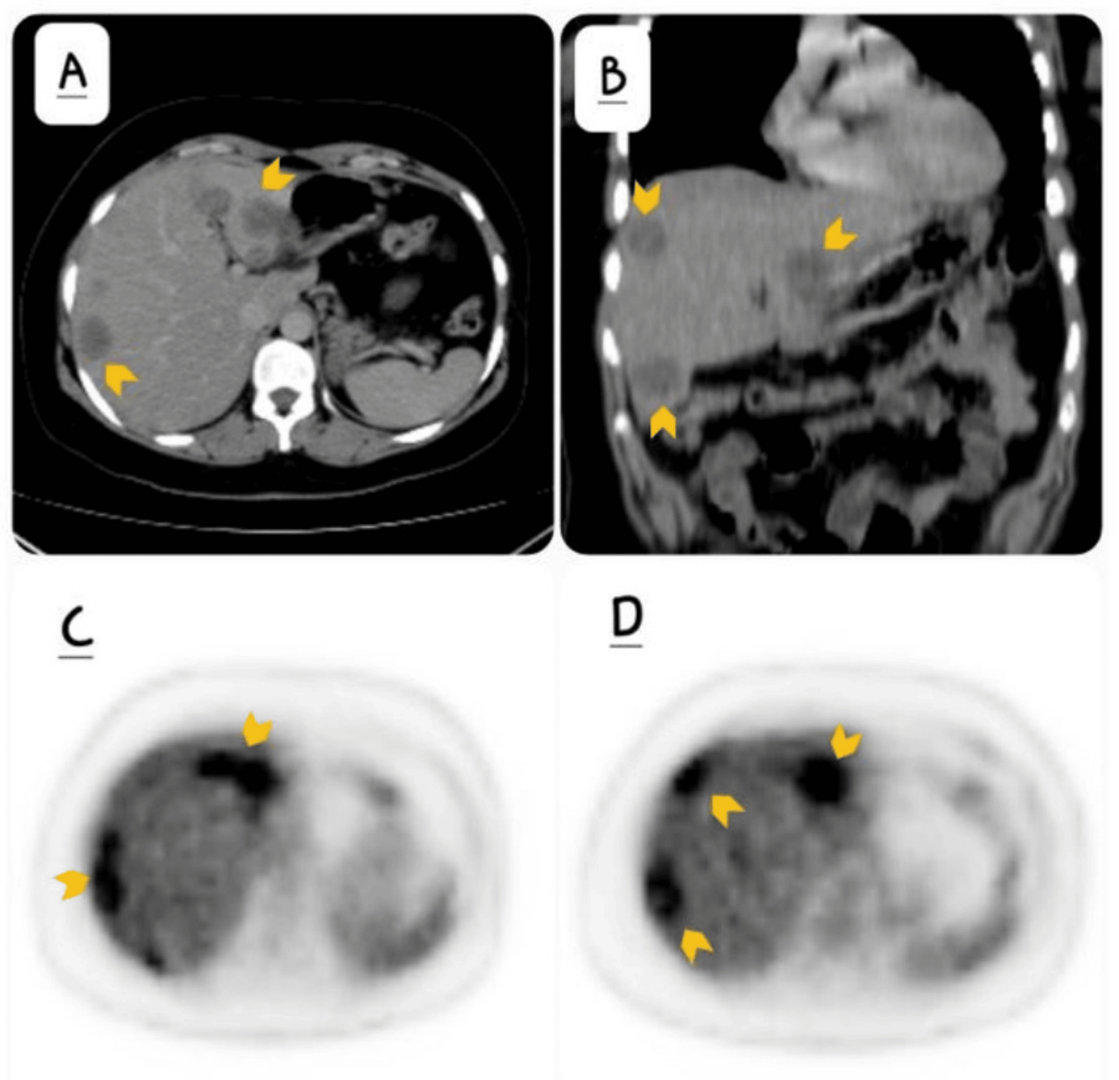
Imaging findings A: axial post-contrast computed tomography (CT) of the abdomen and B: coronal post-contrast computed tomography (CT) demonstrating well-circumscribed hypodense lesions in both lobes of the liver (yellow arrows); C, D: axial fluorodeoxyglucose-positron emission tomography (FDG-PET) confirmed these lesions to be hypermetabolic (yellow arrows).
